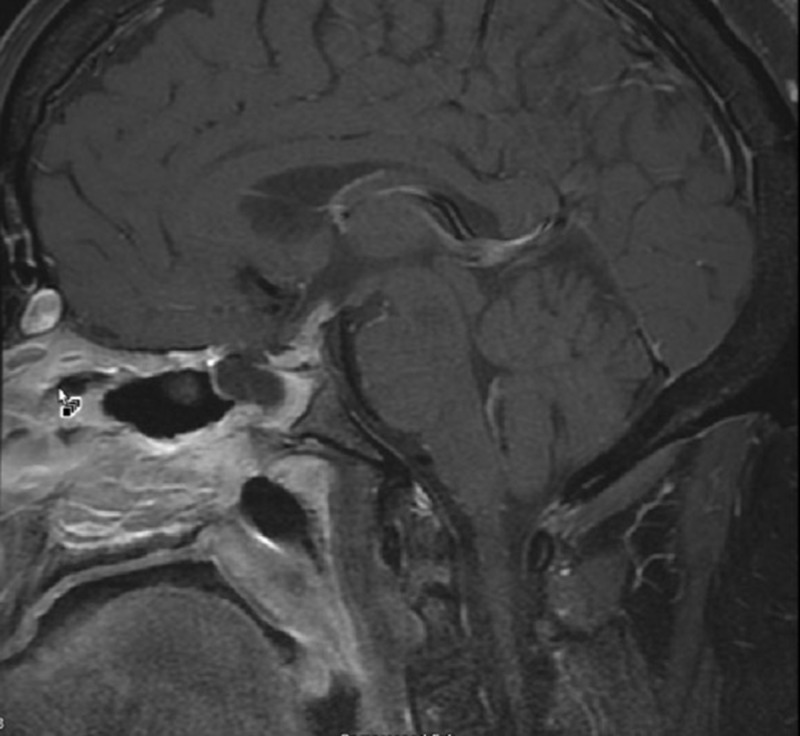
Sagittal T1 (Post-operative) Post-operative brain MRI approximately 8 weeks after surgical resection of the lesion.
